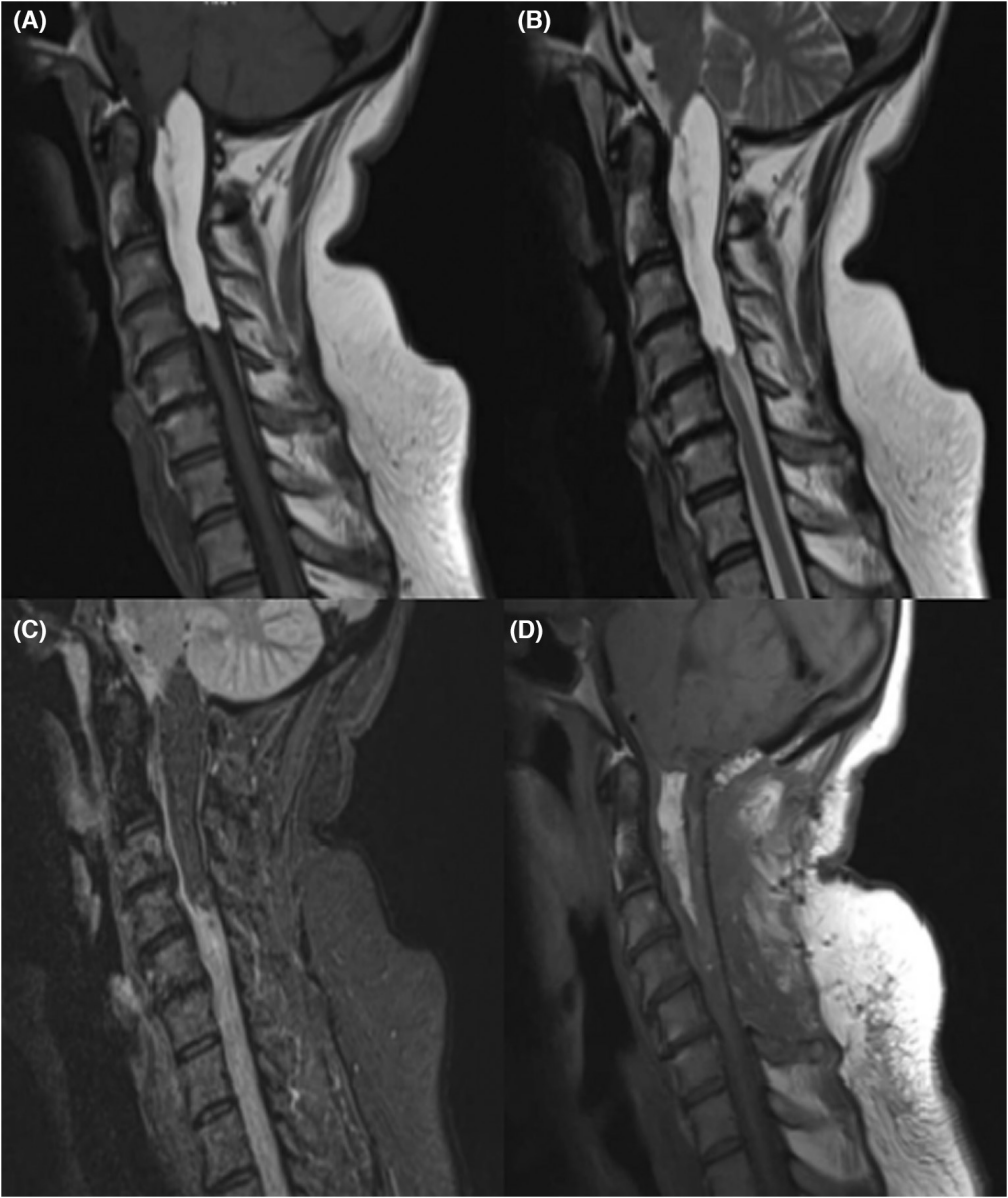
(A) Sagittal T1‐weighted view of the craniocervical region showing hyperintense perimedullary lesion. (B) Sagittal T2‐weighted view of the craniocervical region showing hyperintense perimedullary lesion. (C) Sagittal fat‐suppression sequence of the craniocervical region showing an intensity reduction in the lesion. (D) Post‐operation T1‐weighted MRI is showing subtotal resection of the lesion.
